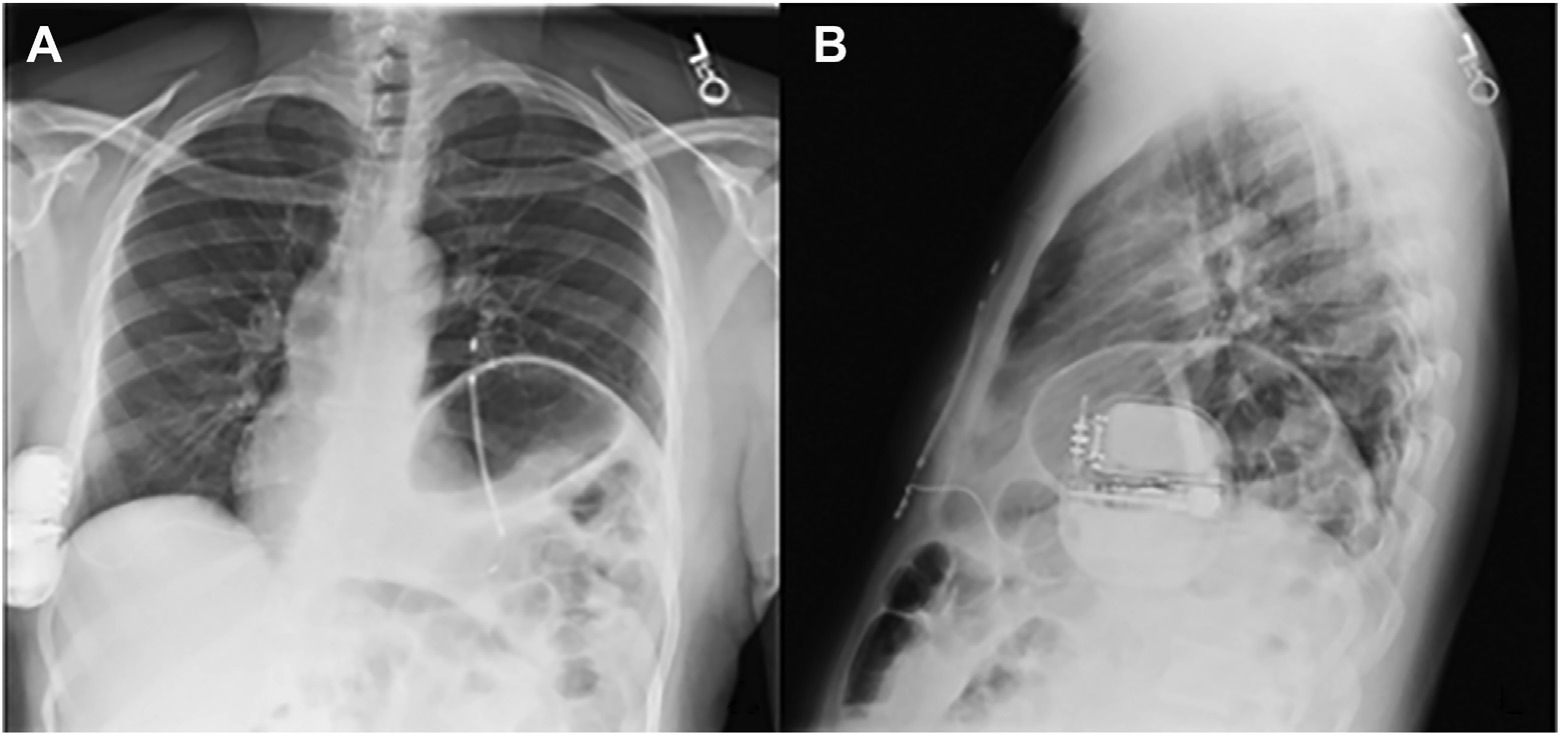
Posteroanterior (A) and lateral (B) plain chest radiographs showing right, lateral thoracic placement of the pulse generator with corresponding left lateral coil placement of the subcutaneous implantable cardioverter-defibrillator. Note the presence of a prominent left hemidiaphragm and associated mesocardia.
